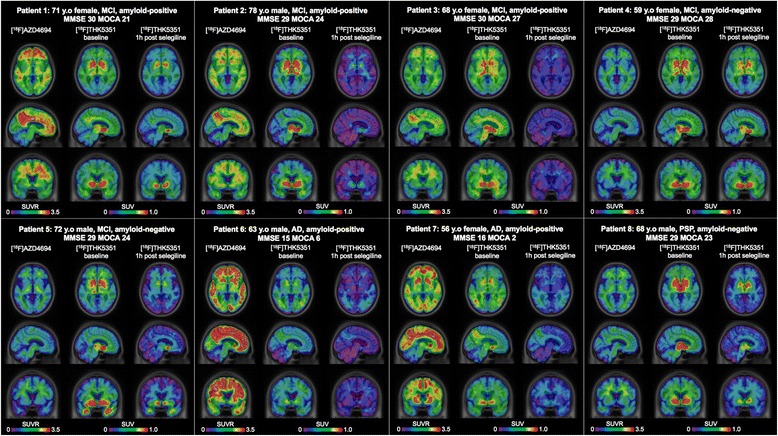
Selegiline reduces brain ^18^F-THK5351 standardized uptake value. Standardized uptake value (*SUV*) and SUV ratio (*SUVR*) map overlaid on a structural MRI scan showing the SUVR from 40 to 70 min after ^18^F-AZD4694 injection, and baseline and 1 h post-selegiline SUV from 50 to 70 min after ^18^F-THK5351 injection in the eight patients. *AD* Alzheimer’s disease, *MCI* mild cognitive impairment, *MMSE* Mini-Mental State Examination, *MoCA* Montreal Cognitive Assessment, *PSP* progressive supranuclear palsy, *y.o* years old
